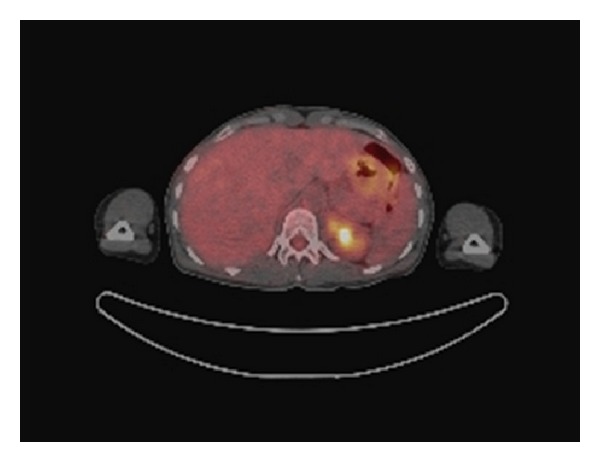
Non-FDG avid liver lesion on PET/CT.
